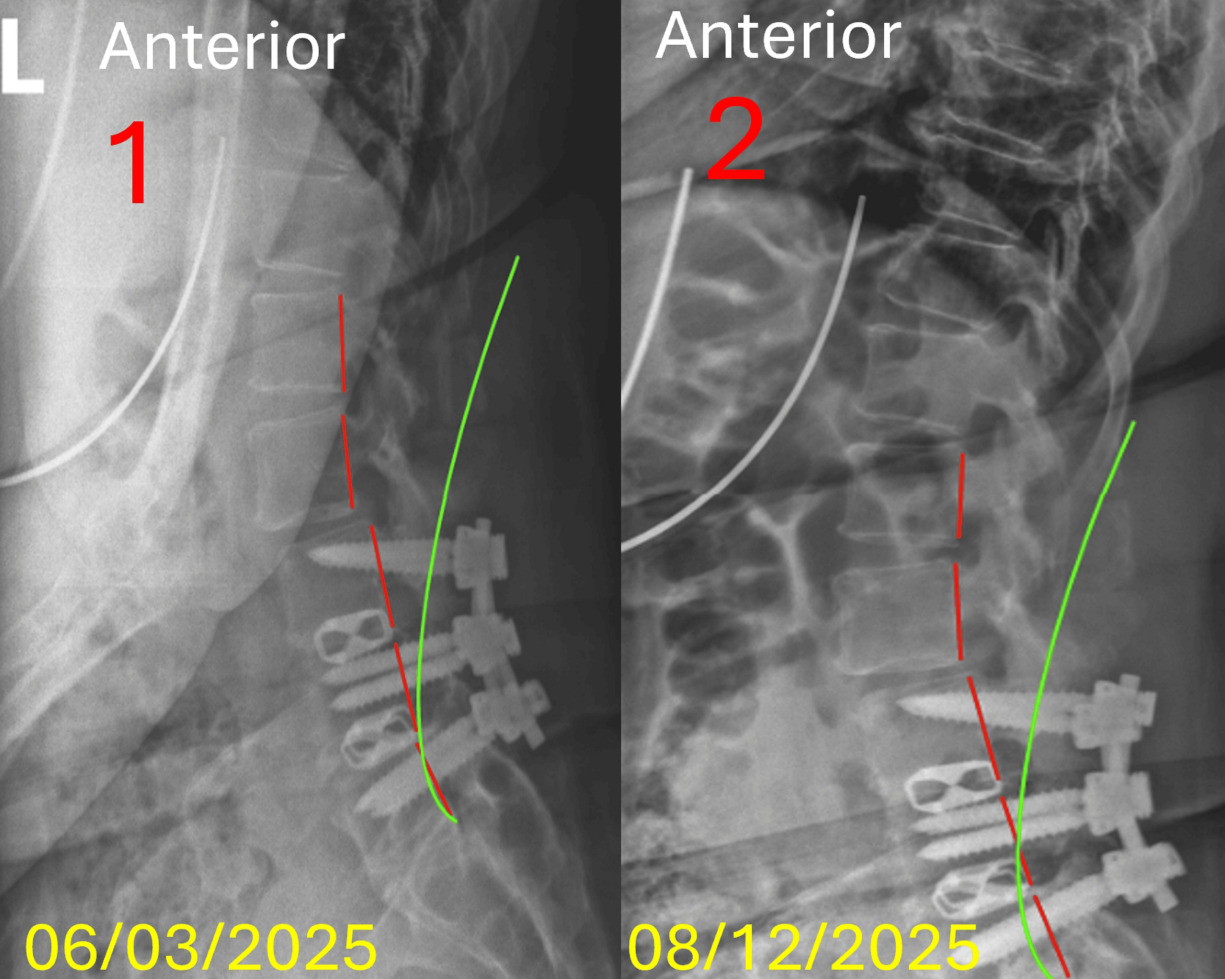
Lateral lumbopelvic radiograph comparison The green line depicts the expected alignment of the posterior longitudinal ligament in a normal spine. The red line represents the subjects' alignment as defined by George’s line, reflecting deviation of the posterior longitudinal ligament. (1) X-ray image represents the initial presentation to the office on 06/03/2025. Pre-treatment neutral lateral lumbar radiograph demonstrating a lumbar curvature (ARA L1-L5) of −27.1 and anterior translation (L1-S1) of 47.7 mm. (2) X-ray image represents the day of discharge from the office on 08/12/2025. Post-treatment neutral lateral lumbar radiograph showing improvement with ARA L1-L5 measuring −24.0 and L1-S1 measuring 30.0 mm. The Absolute Rotation Angle (ARA) tool is a radiographic measurement method used to assess the total angle of spinal curvature.
